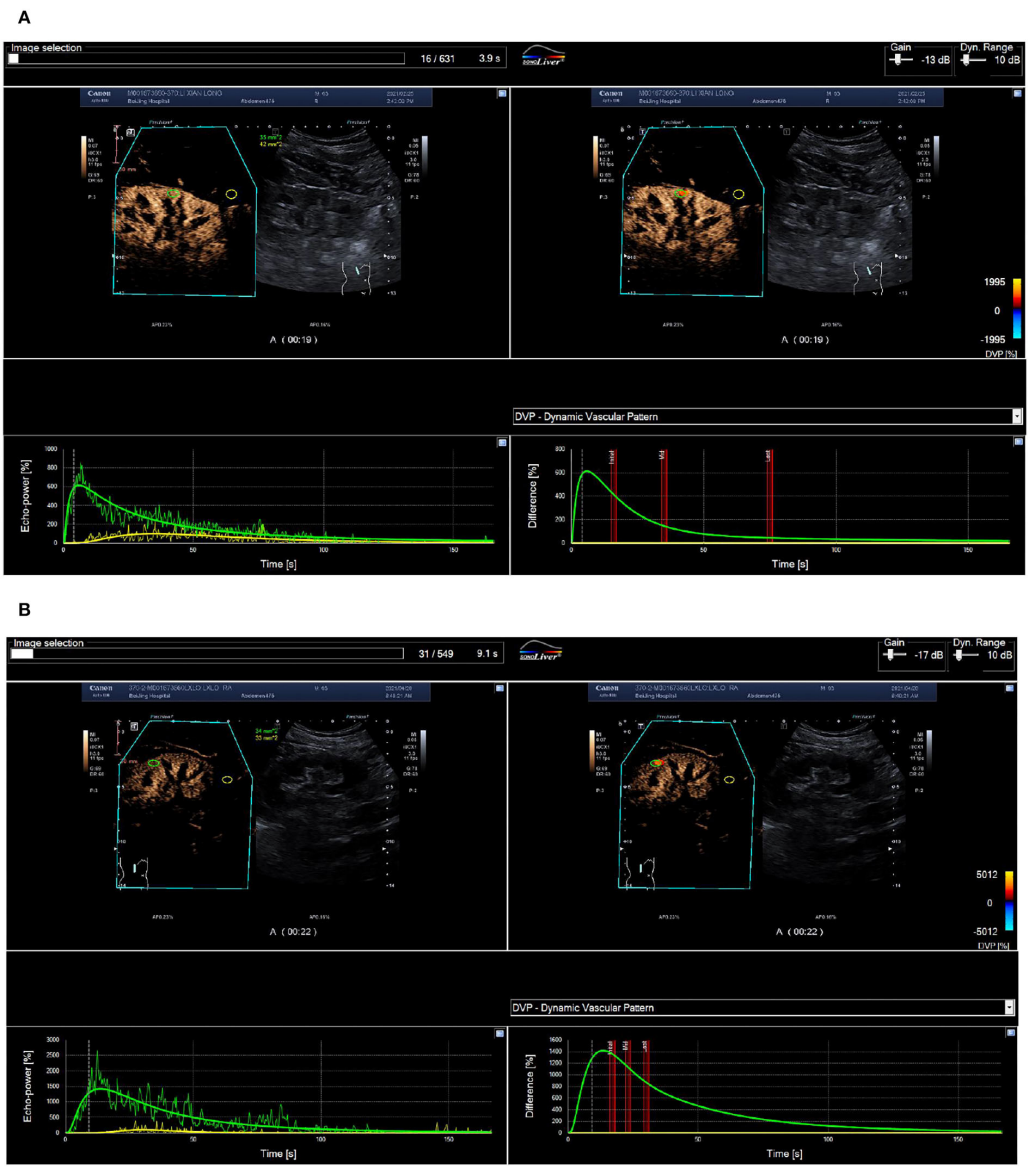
Routine ultrasound and CEUS images of a 65-year-old man with 70% right renal ostial stenosis. (A) Color Doppler flow (left), Doppler frequency spectrum (middle), and CEUS (right) images of the long axis section of right renal artery before PTRAS. (B) After stent implantation, renal artery blood flow images (left) and the peak systolic velocity (middle) of stenosis were corrected, and contrast beam filling (right) displayed normal. RA, renal artery; AO, abdominal aorta. (A) Pre-operative cortical blood perfusion, IMAX (%): 855.1, RT (s): 7.7, TTP (s): 8.3, mTT (s): 42.5, AUC1: 47.5, AUC2: 308.4; (B) Post-operative cortical blood perfusion, IMAX (%): 725.3, RT (s): 6.7, TTP (s): 7.3, mTT (s): 27.9, AUC1: 34.6, AUC2: 194.4. Changes in cortical blood perfusion before (A) and after (B) stent implantation of a 57-year-old woman with severe stenosis of the right renal artery in the BP nonresponse group.
